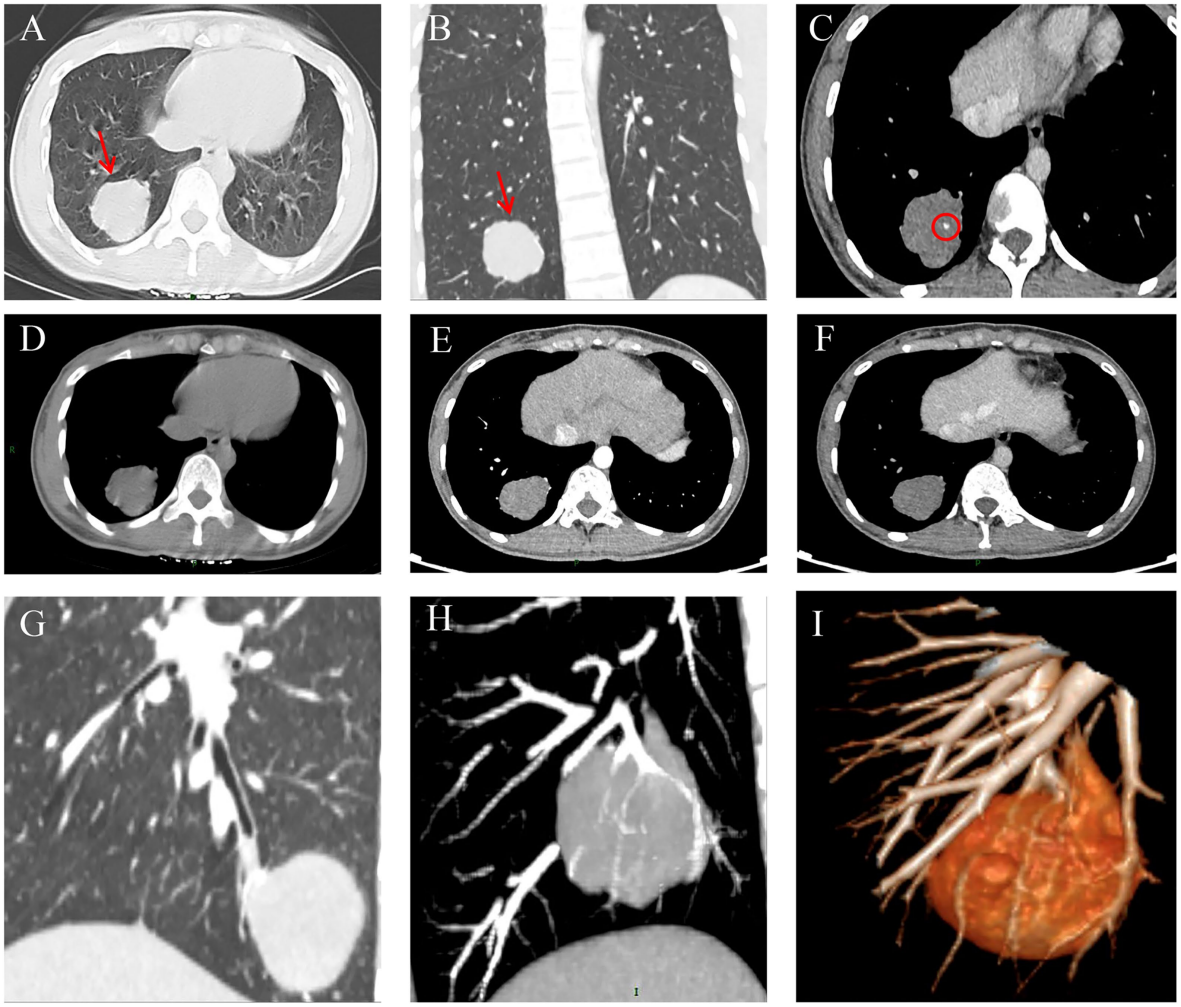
CT images of the patient. The patient’s chest CT shows a spherical soft tissue mass in the right lung’s lower lobe (A,B, red arrow). C and D show uneven density and calcific shadowing, as indicated by the red circle in (C). Lesion enhancement on chest CT showed mild to moderate enhancement with a CT value of 45 HU during the arterial phase (E) and additional enhancement with a CT value of 56 HU during the venous phase (F). No mediastinal lymph nodes were enlarged. MPR showed the lesion was related to the adjacent bronchus (G). MIP (H) and VR (I) showed many vascular shadows around the lesion.
